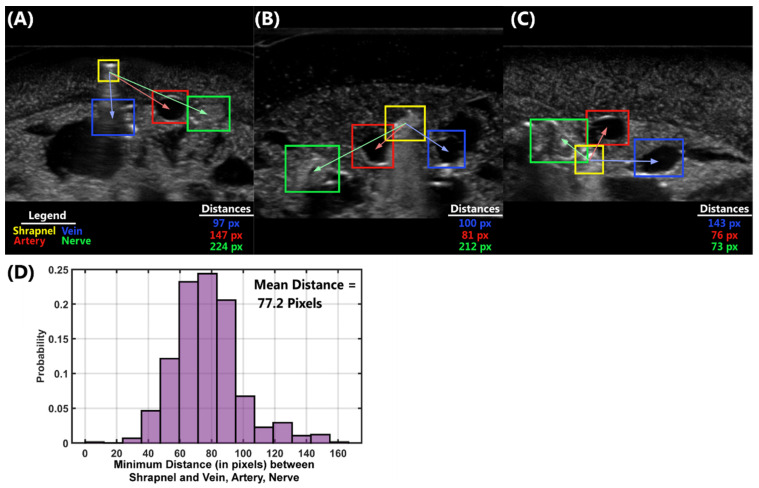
Results for the triage metric determined from the multi-class ShrapOD. (A–C) Distance measurements from center point of shrapnel to each neurovascular feature for three different ultrasound images of varying distances to shrapnel. Only prediction masks are shown (shrapnel = yellow, vein = blue, artery = red, and nerve = green) and distances, in pixels, from shrapnel to the vein (blue), artery (red), and nerve (green) are shown. (D) Probability distribution of minimal distances for each test image containing predictions for all four features. The mean minimal distance across all test images is shown.
